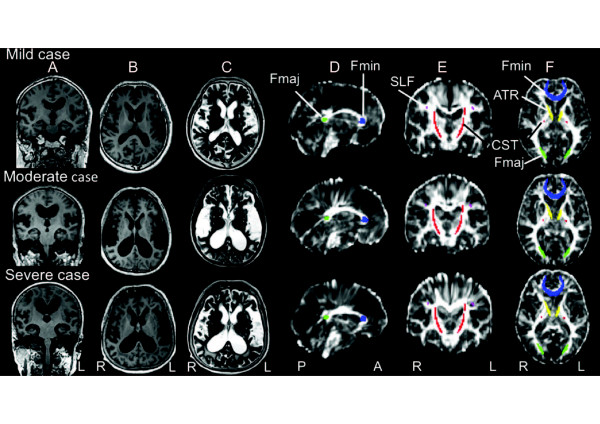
Magnetic resonance images from three idiopathic normal pressure hydrocephalus (INPH) patients arranged in descending order of the anterior thalamic radiation fractional anisotropy (FA) level (mild, moderate and severe). A) T1-weighted image (coronal view). B) T1-weighted image (axial view). C) T2-weighted image (axial view). D) Diffusion tensor image FA map (sagittal view). E) Diffusion tensor image FA map (coronal view). F) Diffusion tensor image FA map (axial view). Regions of interest (ROI) colors: forceps minor (Fmin, blue); anterior thalamic radiation (ATR, yellow); superior longitudinal fasciculus (SLF, purple); corticospinal tract (CST, red); forceps major (Fmaj, green).
